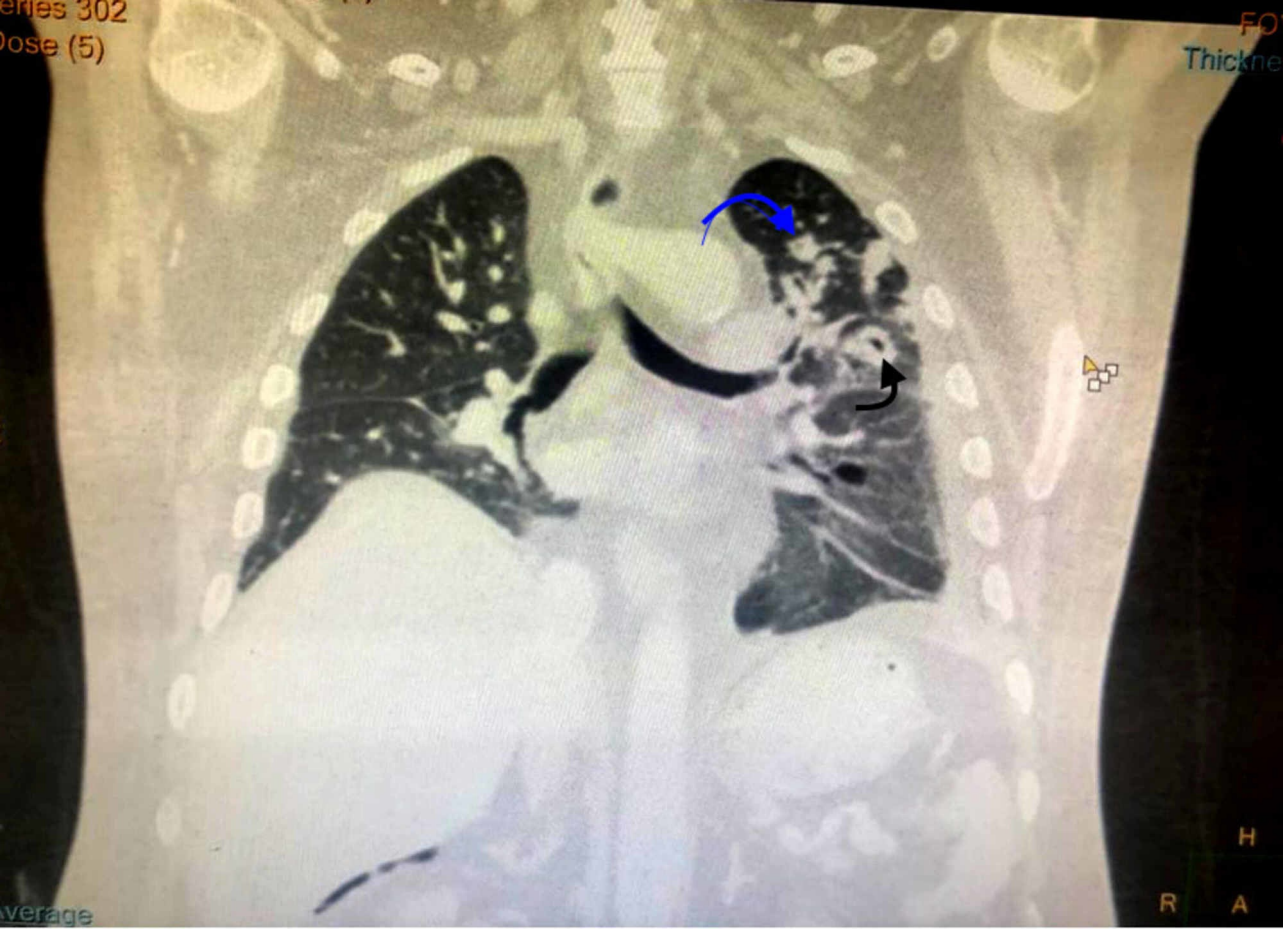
CT scan showing spiculated nodule demostrated by blue arrow and cavitations demonstrated by black arrow.
